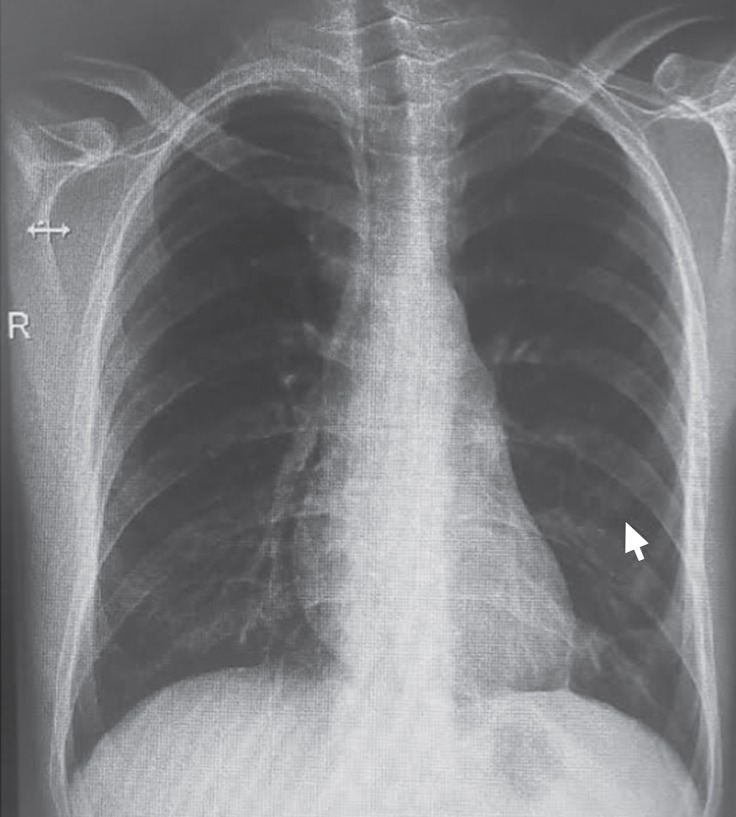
Control posteroanterior radiography, 1 month after the hospital discharge, shows the total resolution of the consolidated region (arrow).
